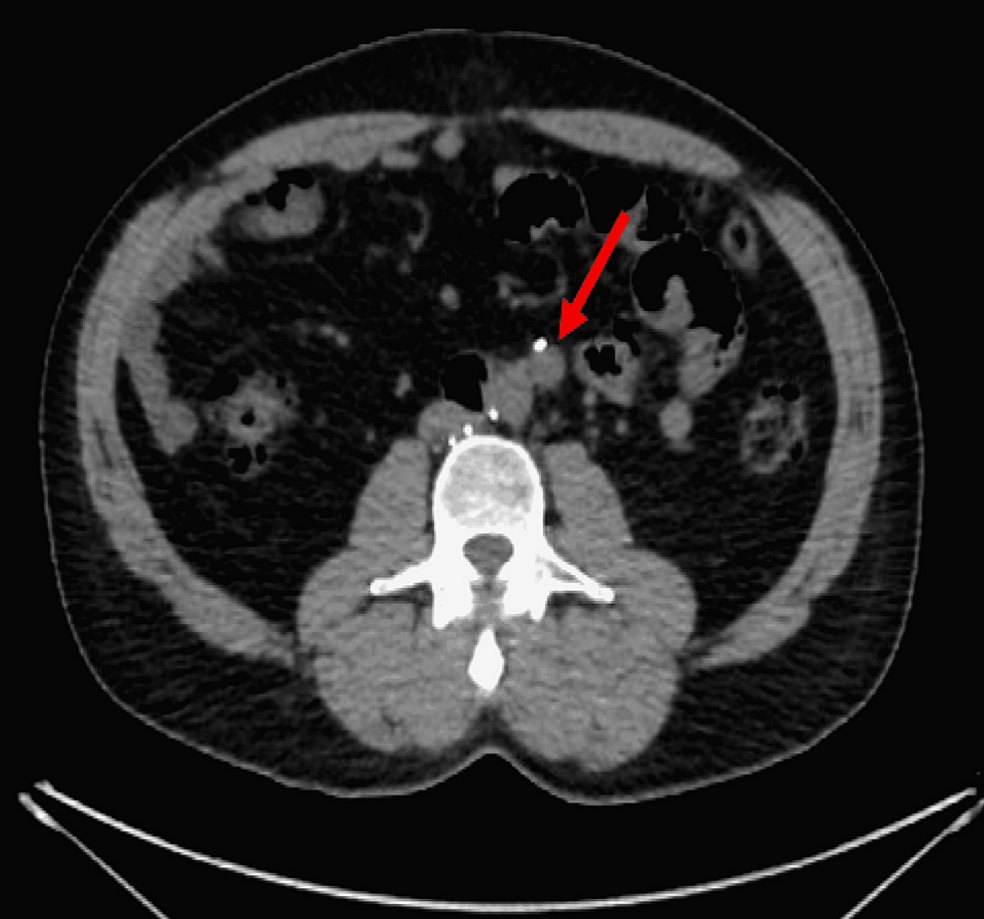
Computed tomography of the abdomen showing an enlarged retroperitoneal lymph node.
